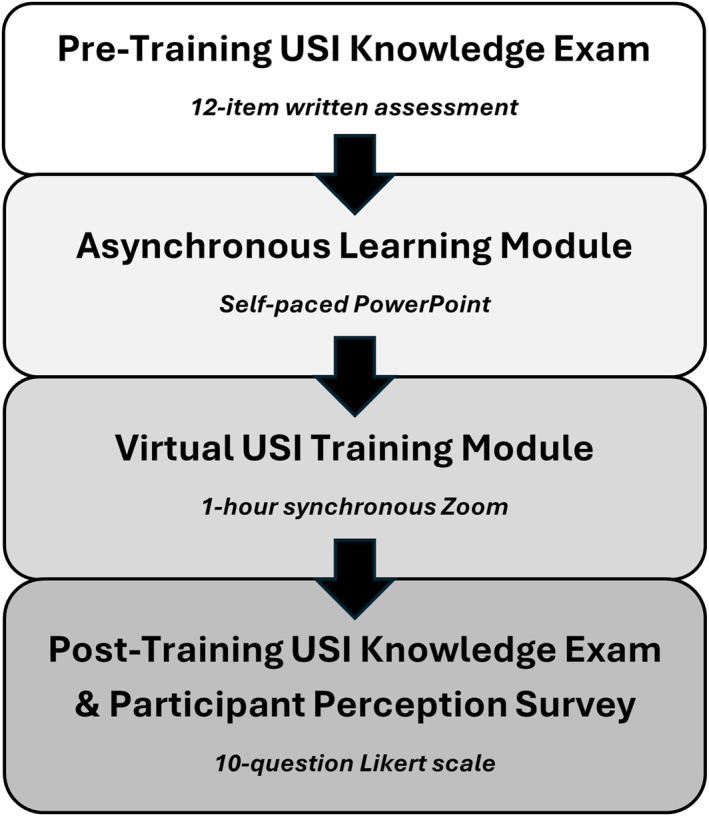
Study workflow for the educational pilot. Participants completed asynchronous pre‐training, a synchronous virtual ultrasound imaging session, and immediate post‐training knowledge and perception assessments.
